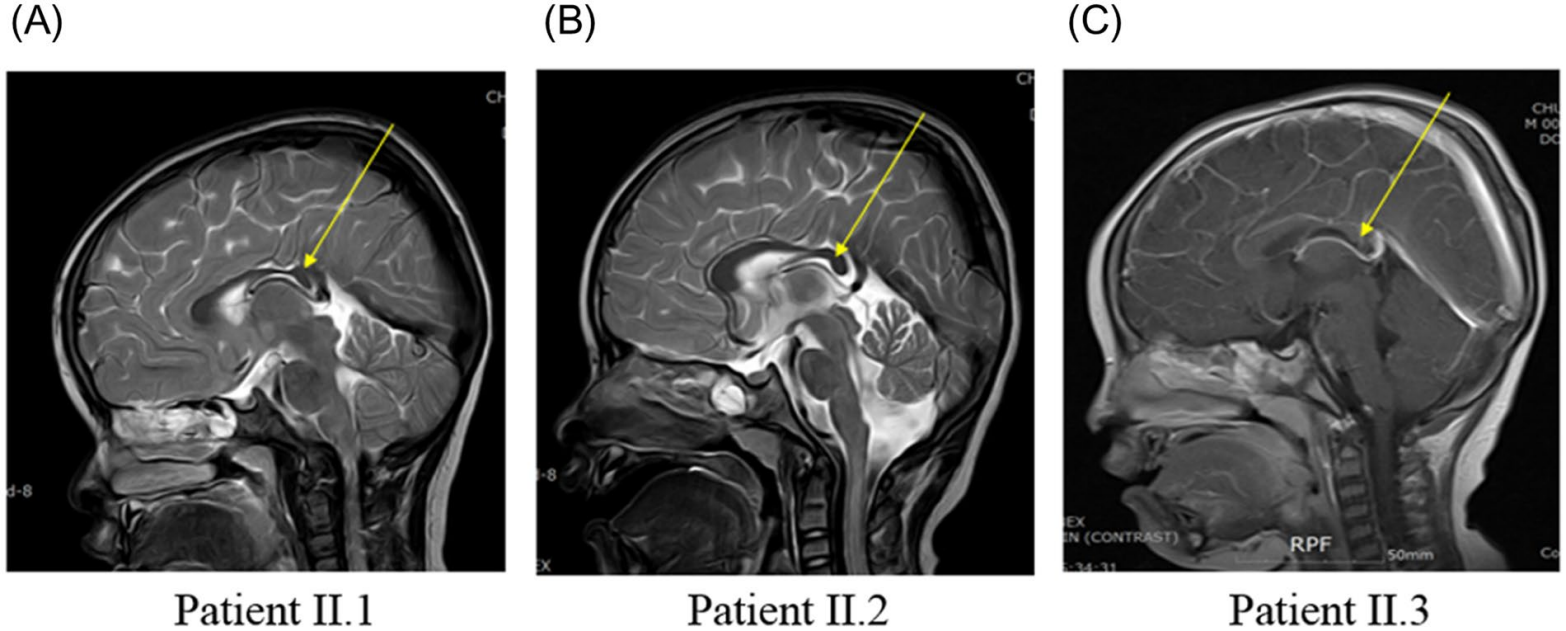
Axial T2-weighted magnetic resonance image of patients. (A, Patient II.1; B, Patient II.2; C, Patient II.3) MRI shows thinning of corpus callosum on axial T2- weighted image which is shown in yellow arrow.
